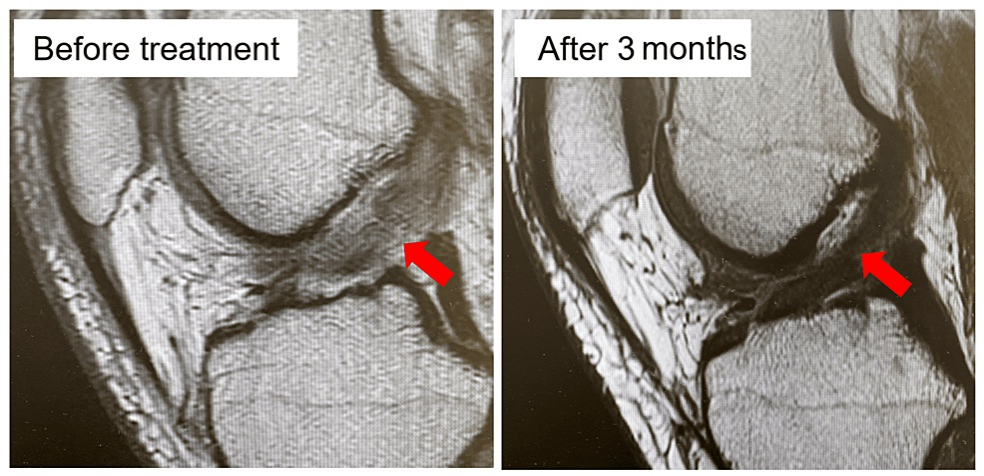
Magnetic resonance imaging of the representative case Case 6: female 33 years old The anterior cruciate ligament, which was torn and disconnected before treatment, has regained continuity three months later, and furthermore, the intra-ligament is considered to have good grade 0 repair with low signal.
